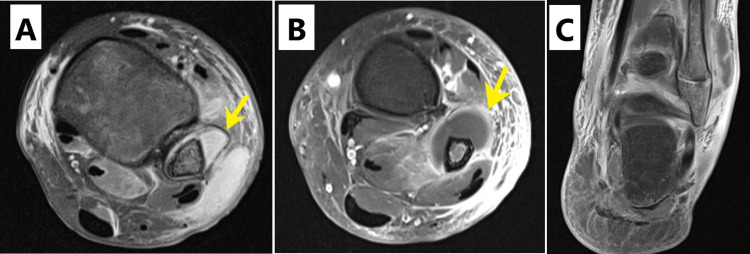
Magnetic resonance imaging (MRI) of the left ankle. (A) Axial proton density fat saturation view demonstrating a subcutaneous and subperiosteal distal fibula abscess (yellow arrow) and surrounding subcutaneous edema. (B) Axial T1-weighted fat saturation view demonstrating the same abscess with peripheral enhancement (yellow arrow) postgadolinium contrast and surrounding subcutaneous edema. (C) Coronal T1-weighted fat saturation, postgadolinium contrast demonstrating subperiosteal abscess at the level of lateral malleolus with surrounding subcutaneous edema.
